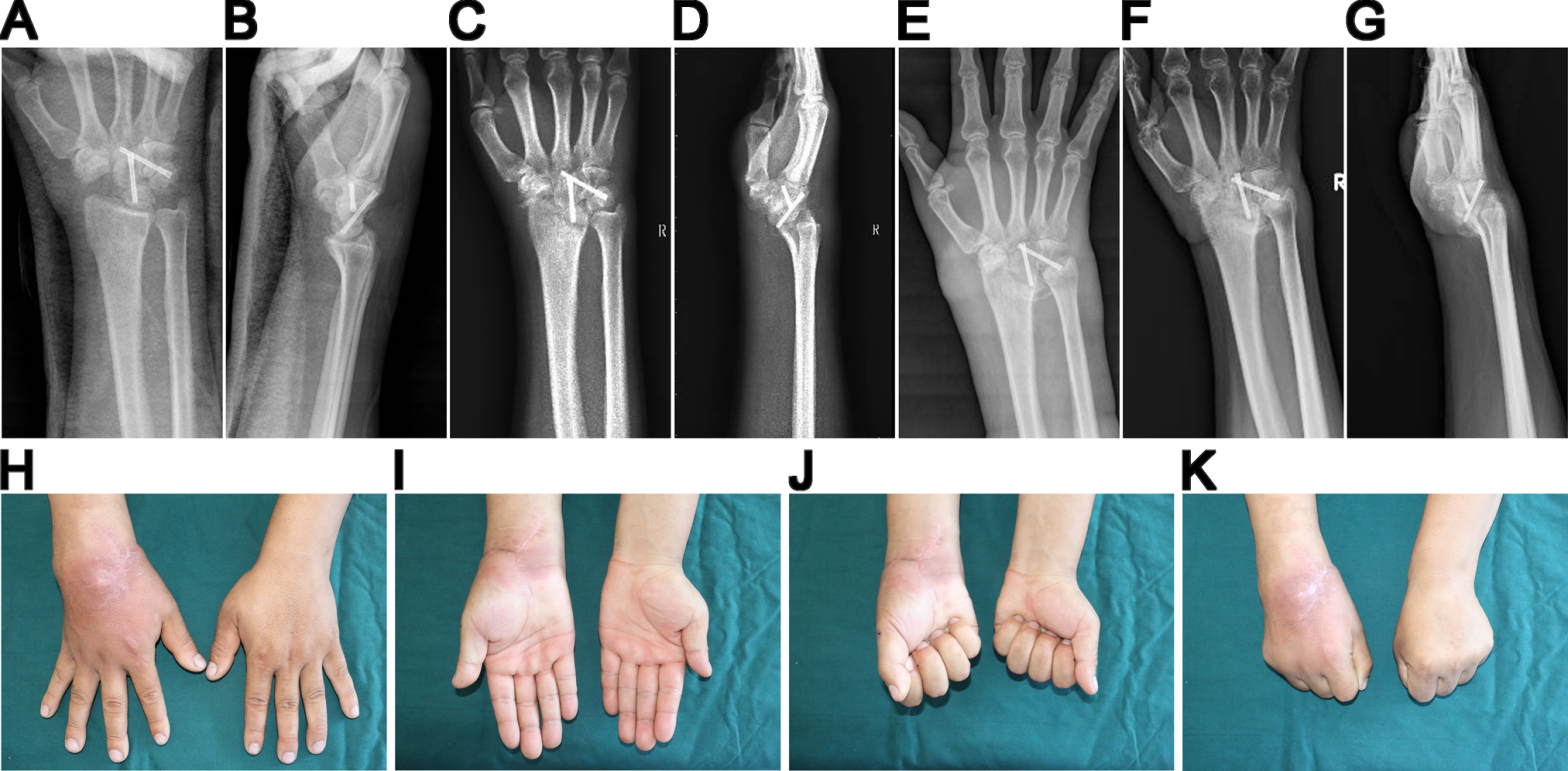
(A) (B) X-ray showing the results of the surgery. (C) (D) Follow-up 1 month after surgery. (E) 2 months after surgery, the swollen wrist and destruction of carpal bone. (F) (G) Follow-up after 103 days, with the right wrist spontaneously and completely fused. (H) –(K) 1 year after the surgery, no wrist pain but with limitation in range of motion.
